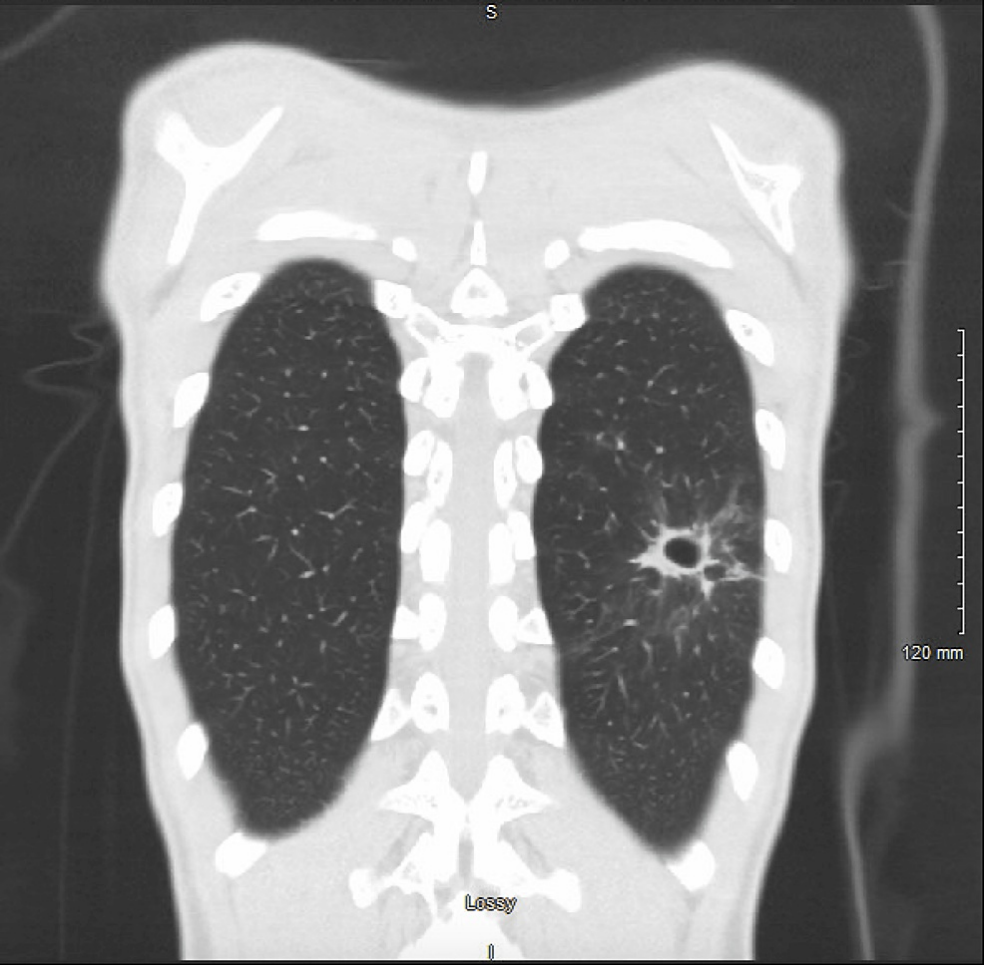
Coronal section of a computed tomography image showing resolution of the lung mass with residual cavitation in its place
